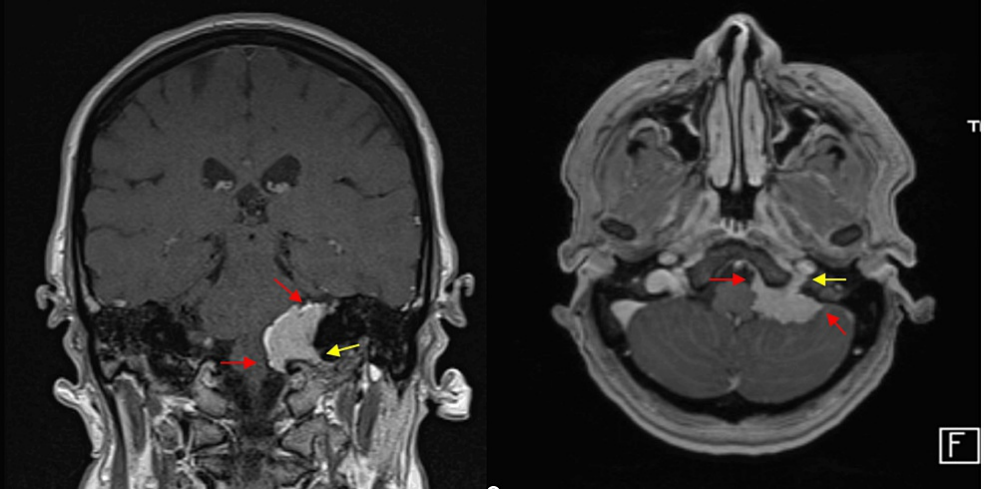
Pre-operative MRI shows a contrast-enhancing lesion in the cerebellopontine angle with a dural tail with brain stem compression (red arrows). An extension to the meatus acusticus internus can be seen (yellow arrows).
